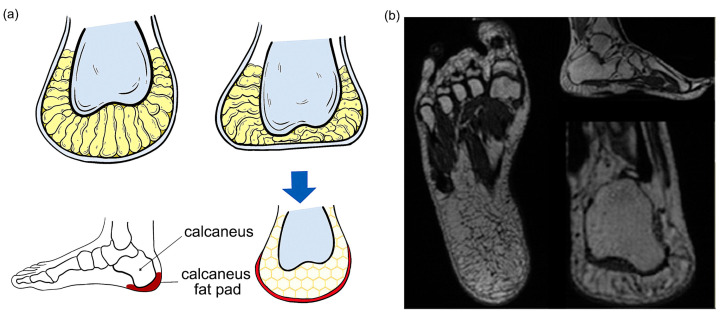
Human heel pad and its magnetic resonance imaging (MRI) images. (a) Indication of nonstress and stress on the heel pad of the normal foot. The position and the honeycomb structure of the heel pad were depicted; (b) MRI image of the human heel pad.
